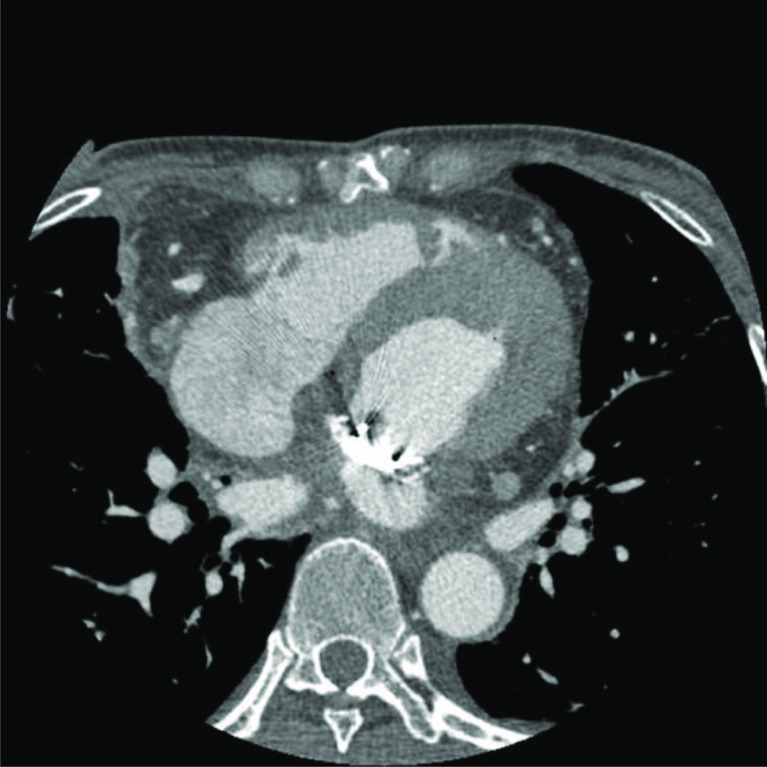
Preoperative contrast CT indicates that omental tissue covers from the right atrium to the entire surface of the aorta. CT: Computed Tomography.
